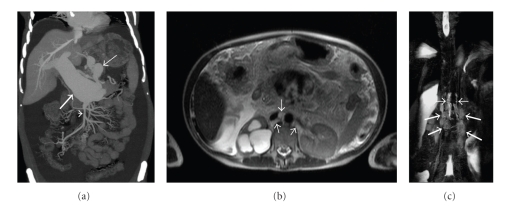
(a) Coronal plane maximum-intensity-projection reformatted CT image shows the fusiform dilatation of the main portal vein (thick arrow). There is also marked dilatation and tortuosity at coronary vein (thin arrow). Note that the caliber of superior mesenteric vein is normal (arrowhead). (b) Axial half-Fourier acquisition single-shot turbo spin-echo (HASTE) MR image demonstrates thick lymphatic channels in the retrocrural space (arrows). Also, there is hydnonephrosis at the right kidney. (c) MR-Lymphangiography image (a half-Fourier single-shot turbo spin-echo 2D sequence with breath-hold technique with maximum intensity projection (MIP)) demonstrates two tortuous tubular structures on each side of the aorta representing dilated cisterna chyli (thin arrows). The meshwork of saccular lymphatic channels in the lumbar region inferior to the cisterna represents the dilated lumbar lymphatics (thick arrows).
